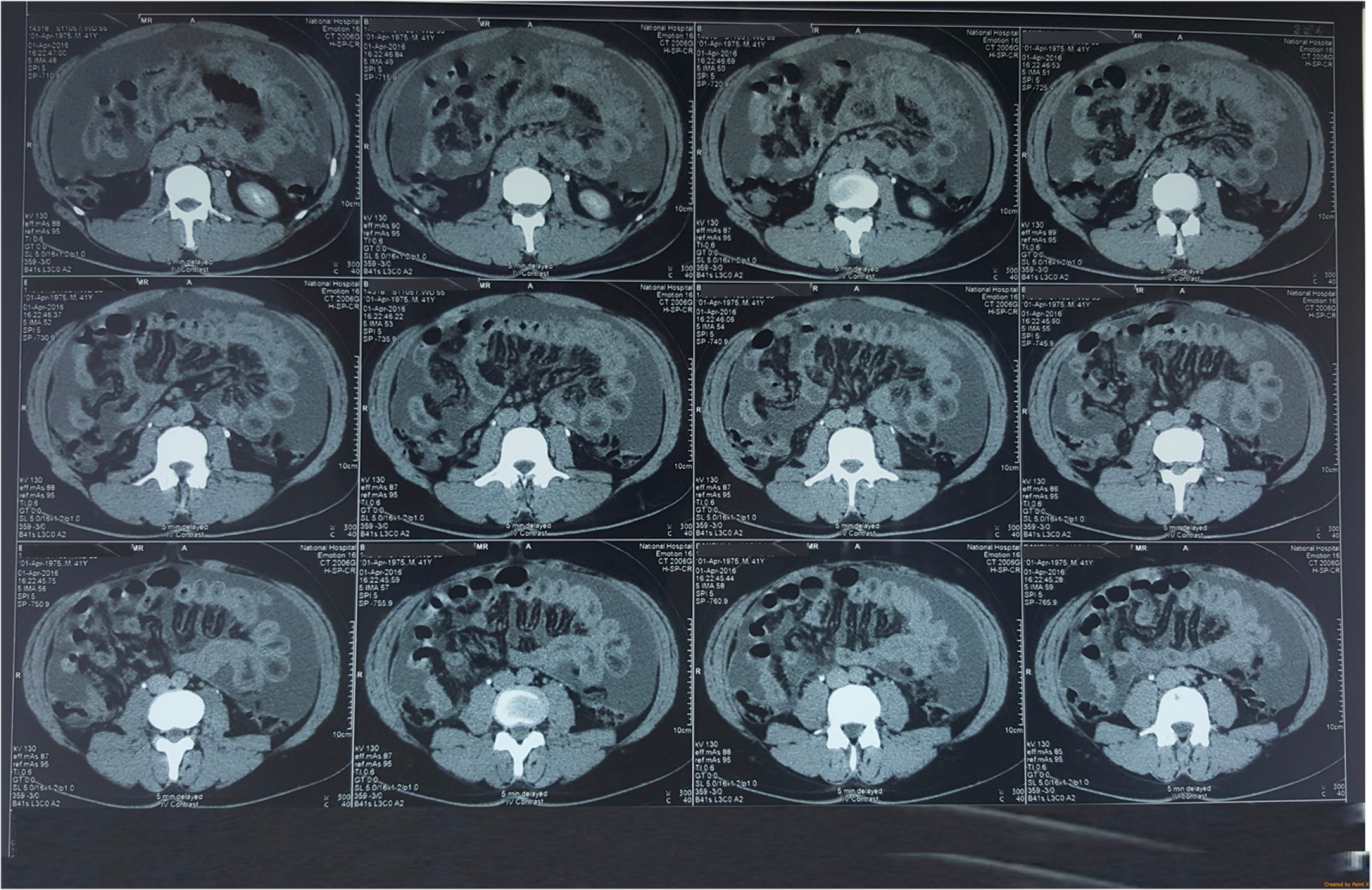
Gross ascites. Contrast-enhanced computed tomography of the abdomen
